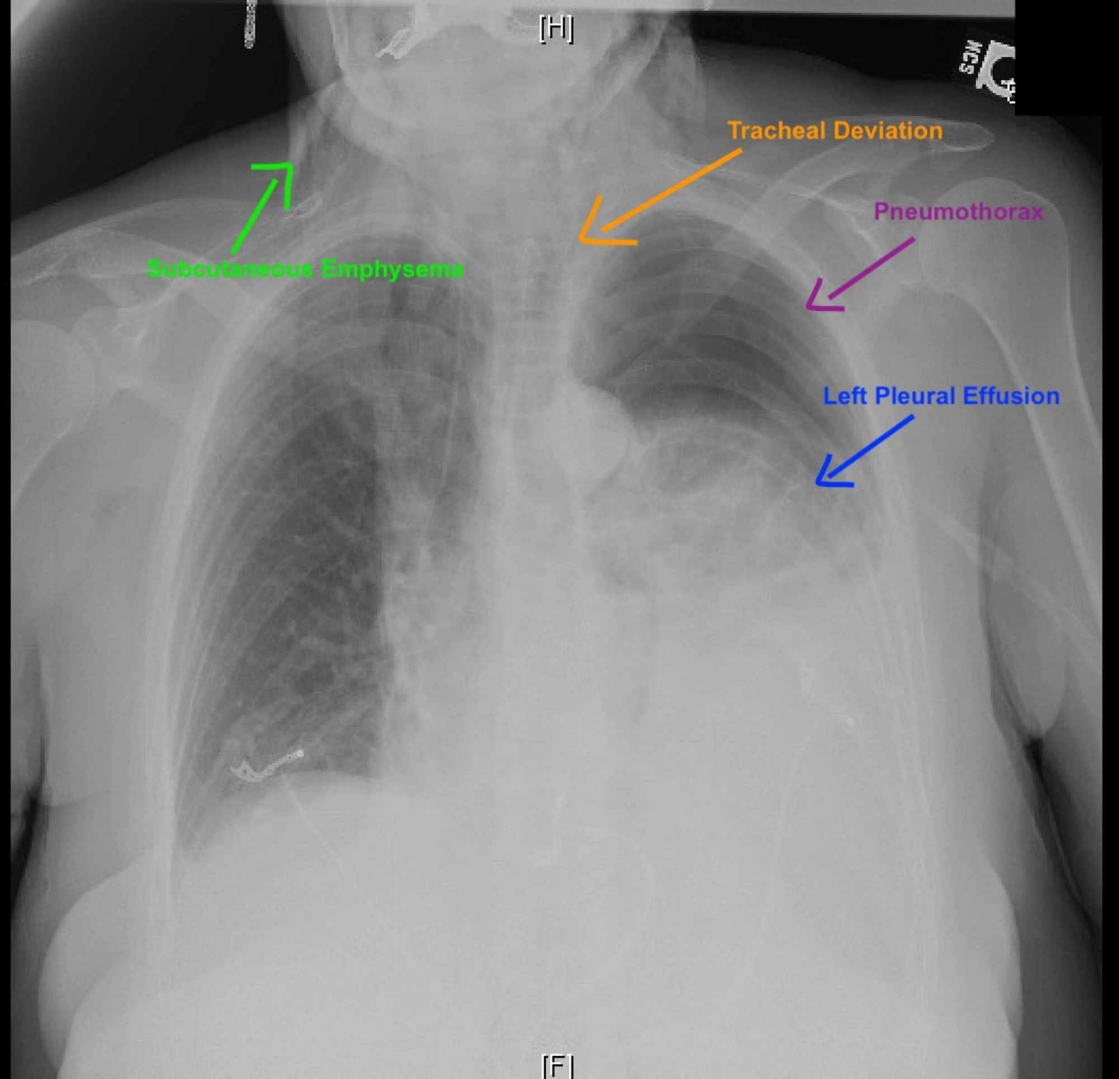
Chest radiograph demonstrating pneumothorax, pleural effusion, tracheal deviation, and subcutaneous emphysema
